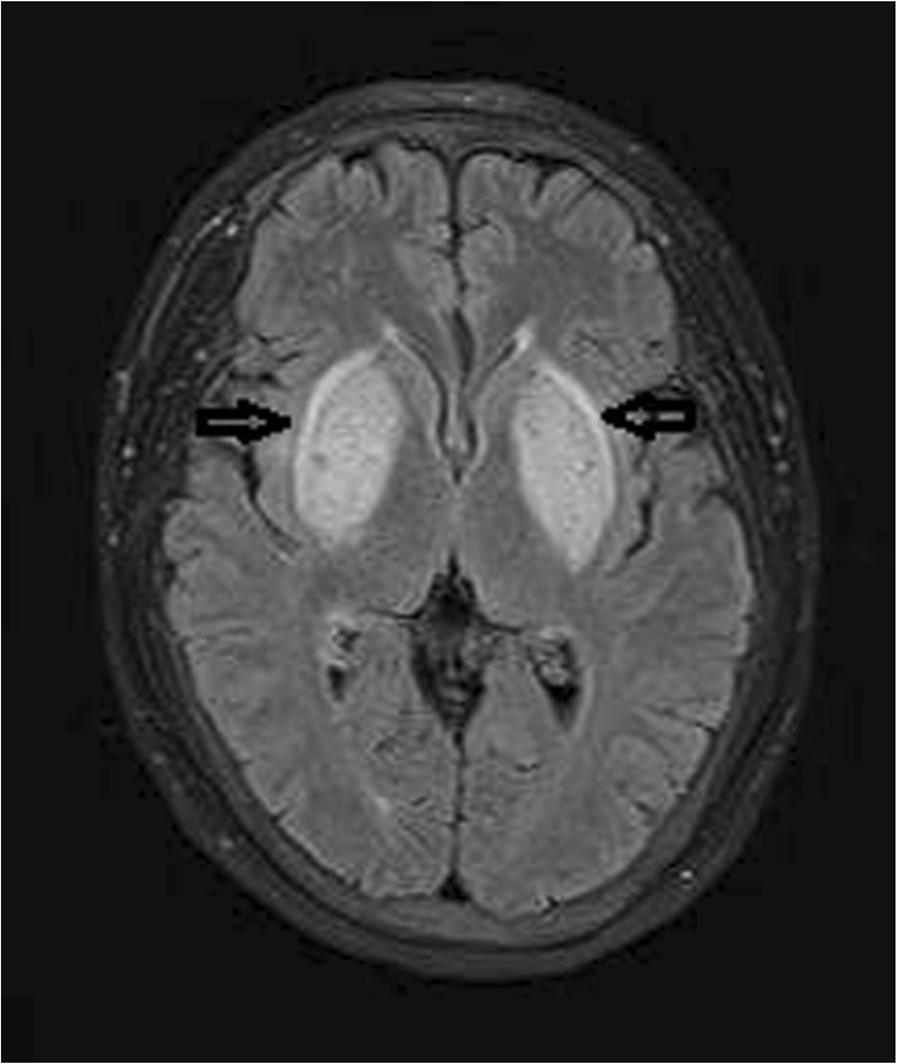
Brain MRI performed upon admission. T2FLAIR showed symmetrical hyperintense non-hemorrhagic lesions in the entire bilateral basal ganglia (arrows) and corona radiata lesions showing mild diffusion restriction
